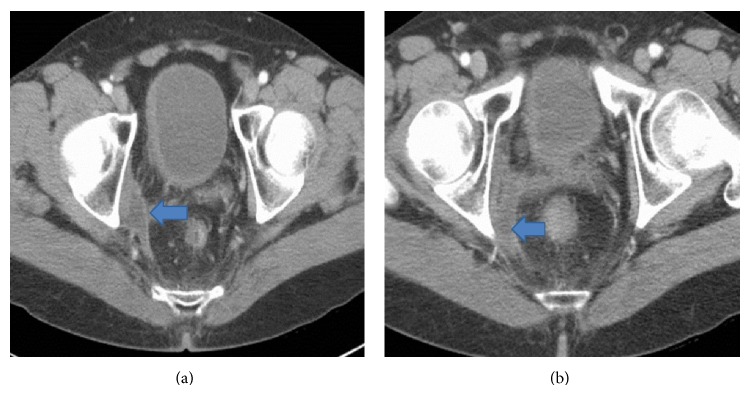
Contrast-enhanced CT scan of the pelvis demonstrates a 3.4 × 1.7 cm pelvic lesion (arrows) with no substantial soft-tissue component. The lesion is linear and elongated and appears to be comprised mainly of necrotic tissue, consistent with postradiation changes.
